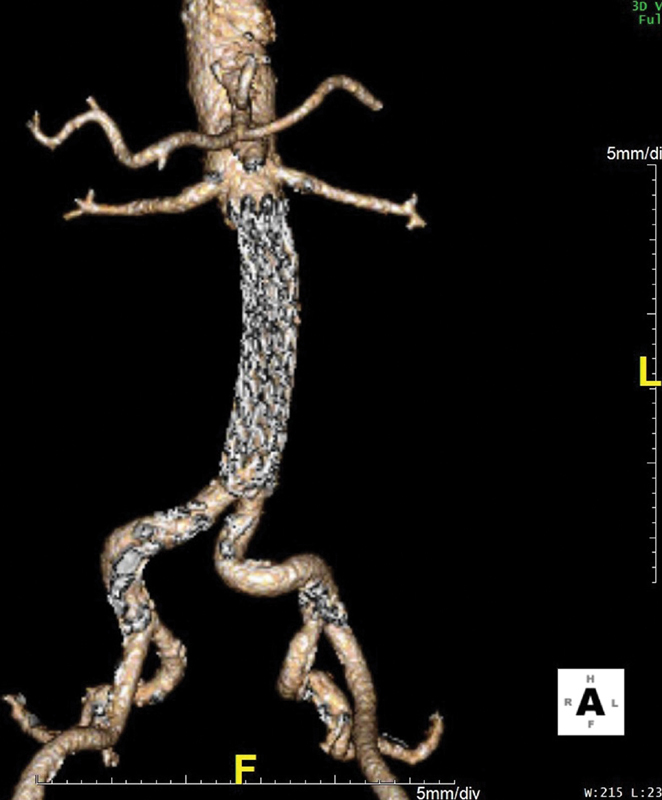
Computed tomography angiography at 37-day follow-up revealed no active extravasation or endoleak. The hematoma was reduced by 2 cm compared with the previous computed tomography.
